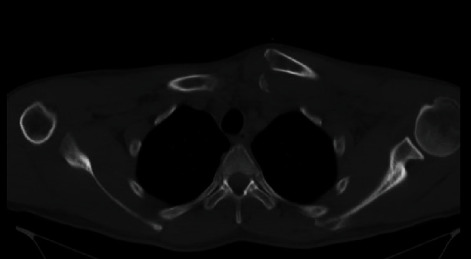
Axial CT scan demonstrating anteriorly displaced fracture dislocation of the SC joint.
